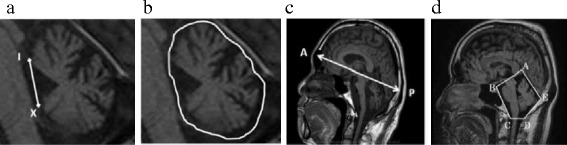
Segmentation of the cerebellum on MR images. The cerebellum was defined as the area lateral to the line connecting the anterior lobe of the cerebellum (I segment) and cerebellar flocculus (X segment) (a). Using DICOM data from cranial MRI T1 sagittal sections, the cerebellum was traced freehand on each cerebellum MRI sagittal section slice (b). Then, on the central slice, the fourth ventricle and surrounding cerebellar tissue were selected, and the cerebellum was automatically extracted. The cerebellar volume was determined by linear interpolation from the automatically extracted cerebellar tissue area and slice thickness. The anteroposterior diameter of the cranium (the distance between two points, A and P, at which the skull and the anterior commissure-posterior commissure line intersect) was used for the cerebellar volume correction (c). The posterior cranial fossa volume was determined by five anatomical landmarks (A: the upper tentorial end, B: infundibular recess, C: basilar impression, D: posterior pole of the hard palate, E: internal occipital protuberance) and used for additional cerebellar volume correction (d)
